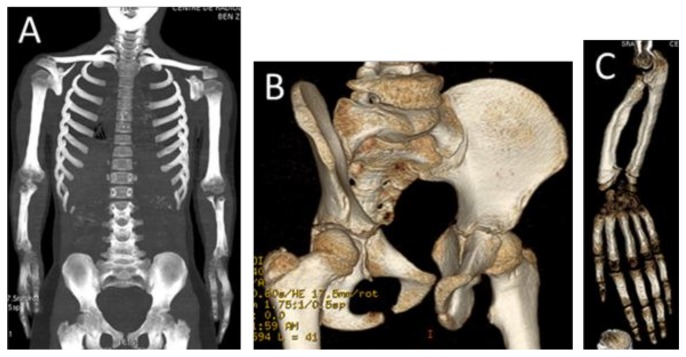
Reconstruction CT scan of the whole skeleton showed rhizomelia, metaphyseal widening of the humeri and a deficient number of ribs (10 instead of 12) (A). Pelvic bones showed coxa valga and iliac–ischial dysplasia due to defective ossification of the ischial rami (B). The forearms showed Madelung´s deformity (short radius and radial dislocation with lateral and posterior bowing, a triangular epiphysis due to absence of the growth plate medially, and posterior dislocation the lower end of the ulna), the carpal bones were wedged between the distal ends of the radius and protruding ulna (C).
